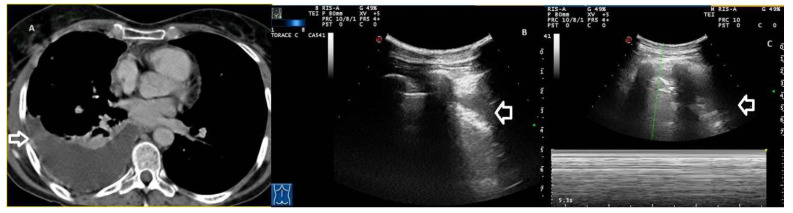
(A) Axial CT scan shows right pleural effusion with a slight thickening of the parietal pleura and an associated basal parenchymal hypoventilation suggestive of pleural empyema (arrow). (B) Transthoracic ultrasound scan with a convex probe (7 MHz) corresponding to the pleural empyema in the CT image, which shows a hyperechoic pleural effusion (arrow). Videoclip 3 also shows the absence of a gliding sign. (C) Transthoracic ultrasound scan in M-mode shows the “bar-code” sign (absence of movement) (arrow).
